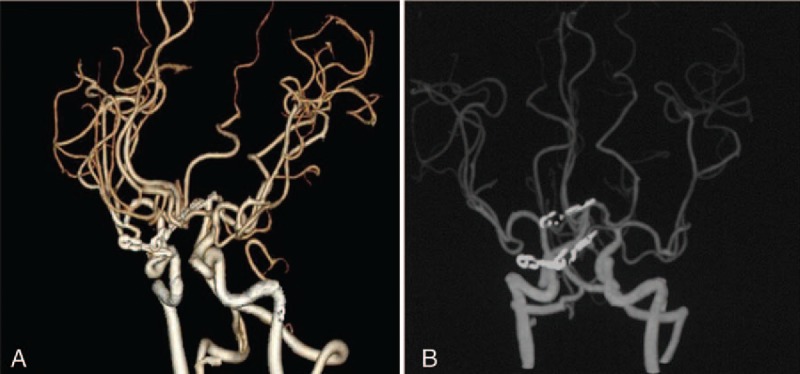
Postoperative CTA showing clipped aneurysms. CTA = computed tomographic angiography.
